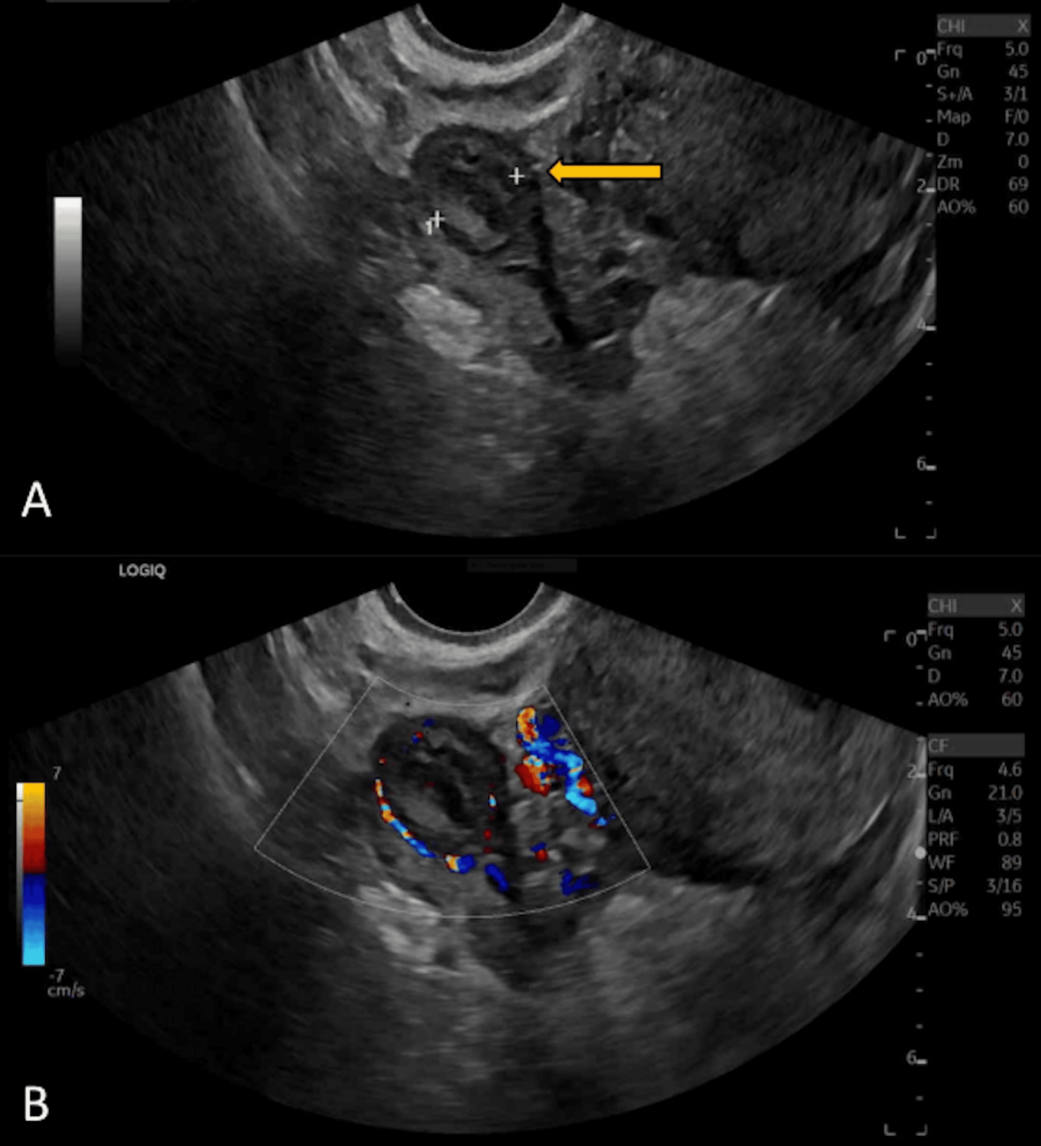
Transvaginal Doppler duplex ultrasound of the right adnexa. Figure 1A depicts a grey-scale image of a 2 x 1 cm hyperechoic area near the right ovary (yellow arrow). Figure 1B demonstrates a spectral and color Doppler image of the right ovary with a circumferential peripheral blood flow pattern characteristic of the “ring of fire” sign (white outline).
